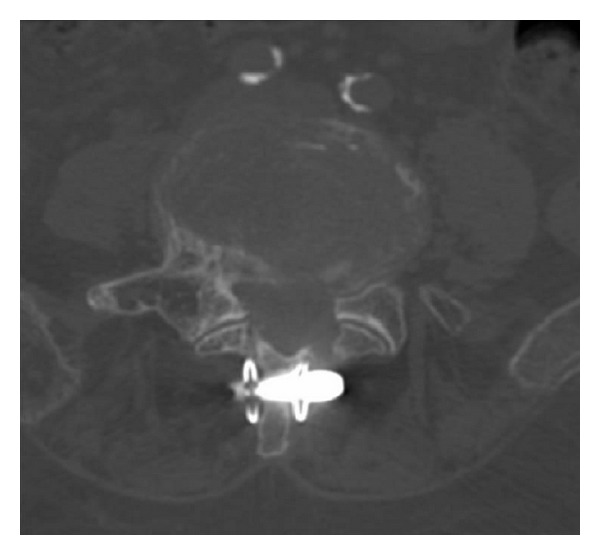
Axial CT image of the Aperius inserted at L4-L5 level showing the wings expanded on each side of the spinous process.
